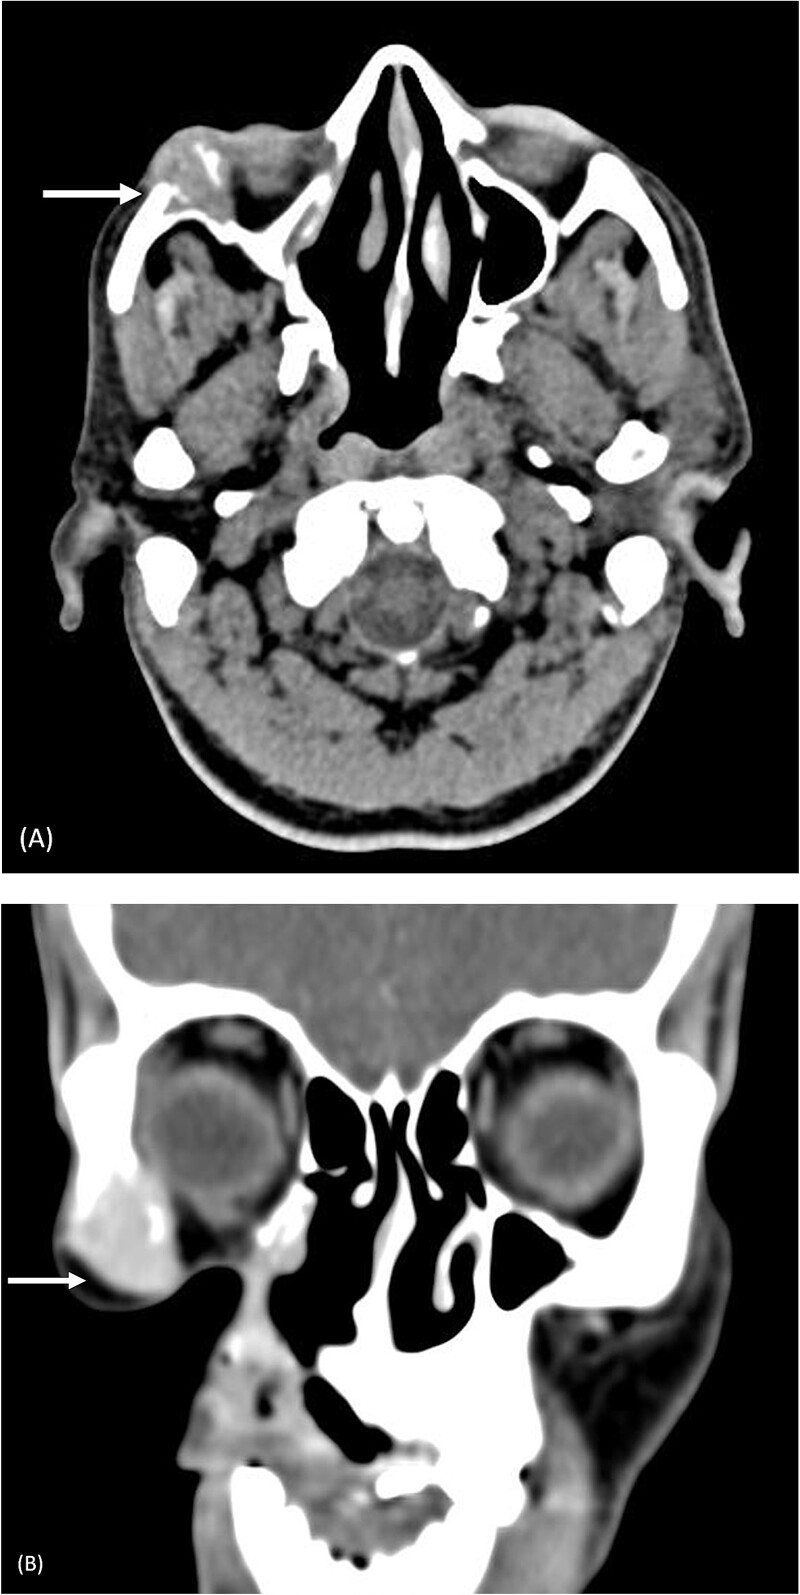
Pre-operative computed tomography of the zygomatic region. (A) Axial CT (bone algorithm) demonstrating a mass of size 2.2 × 2.1 × 2.1 cm^3^ in the right infraorbital region; (B) coronal CT (bone algorithm) demonstrating erosion of the lateral wall of the right orbit and right zygomatic bone, with involvement of the lateral rectus muscle.
